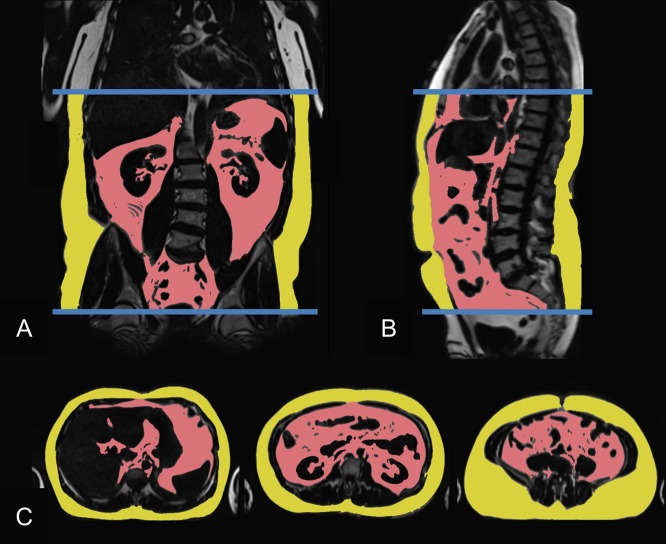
MRI-based assessment of visceral (VAT; red) and subcutaneous (SAT; yellow) adipose tissue in a 46-year-old male (VAT 6.57 l), displayed in coronar (A), sagittal (B) and axial (C) slices.
